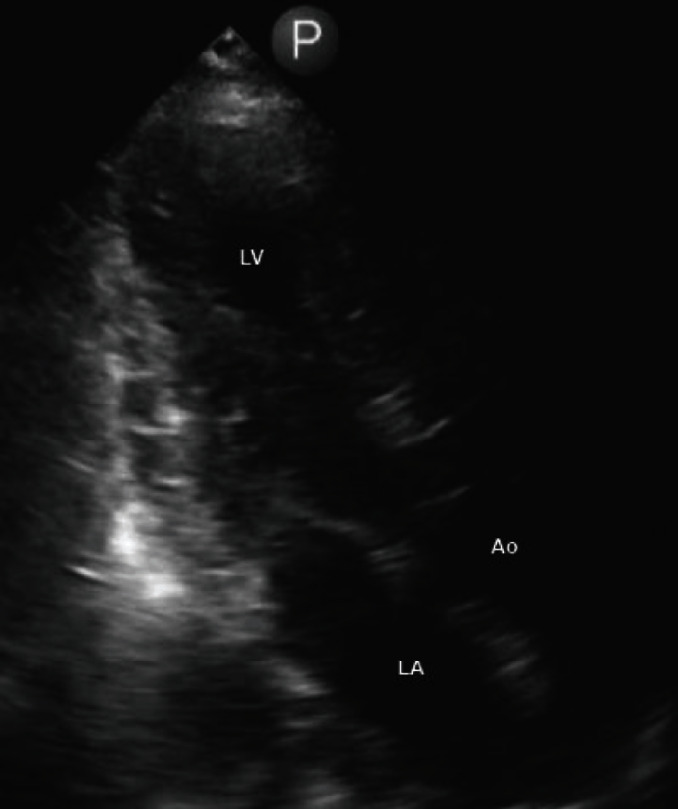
Image of the apical view of transthoracic echocardiogram showing apical hypokinesis.
